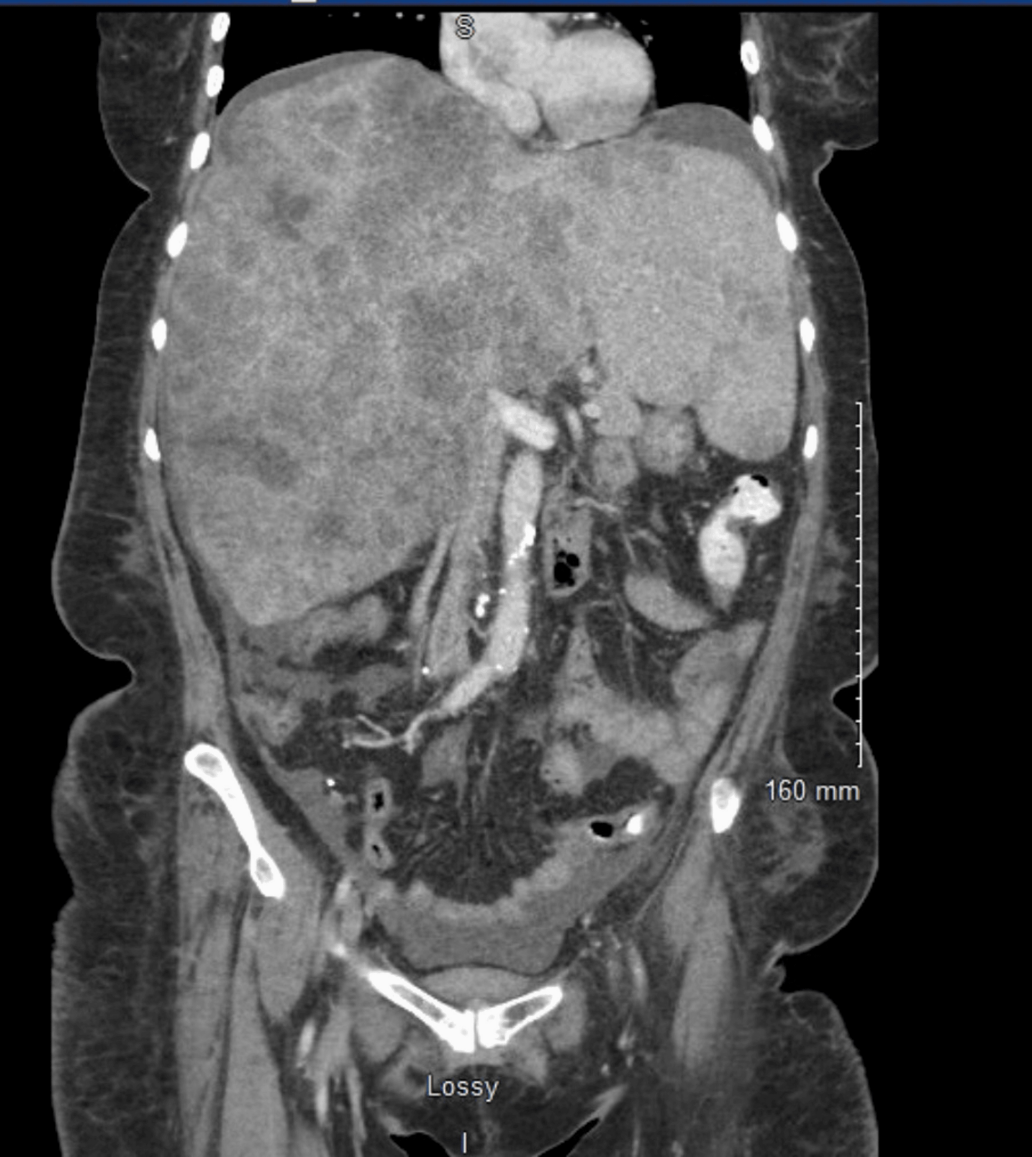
Coronal view of CT abdomen and pelvis showing hepatic densities consistent with the patient’s history of stage IV cervical cancer with hepatic metastases and small-volume ascites.
